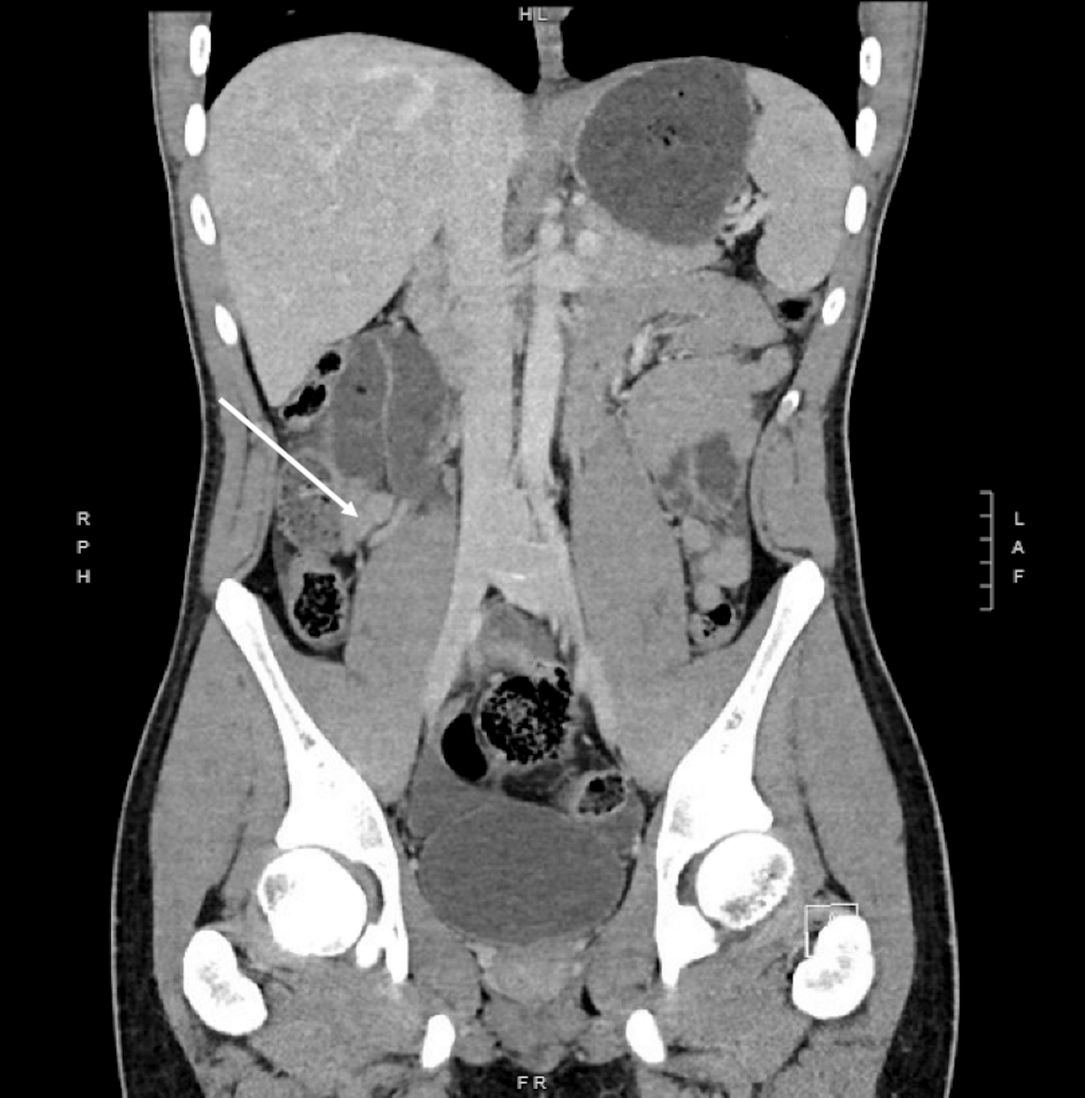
Noninflamed appendix on CT imaging Coronal CT image with arrows indicating noninflamed appendix. CT: Computed tomography
